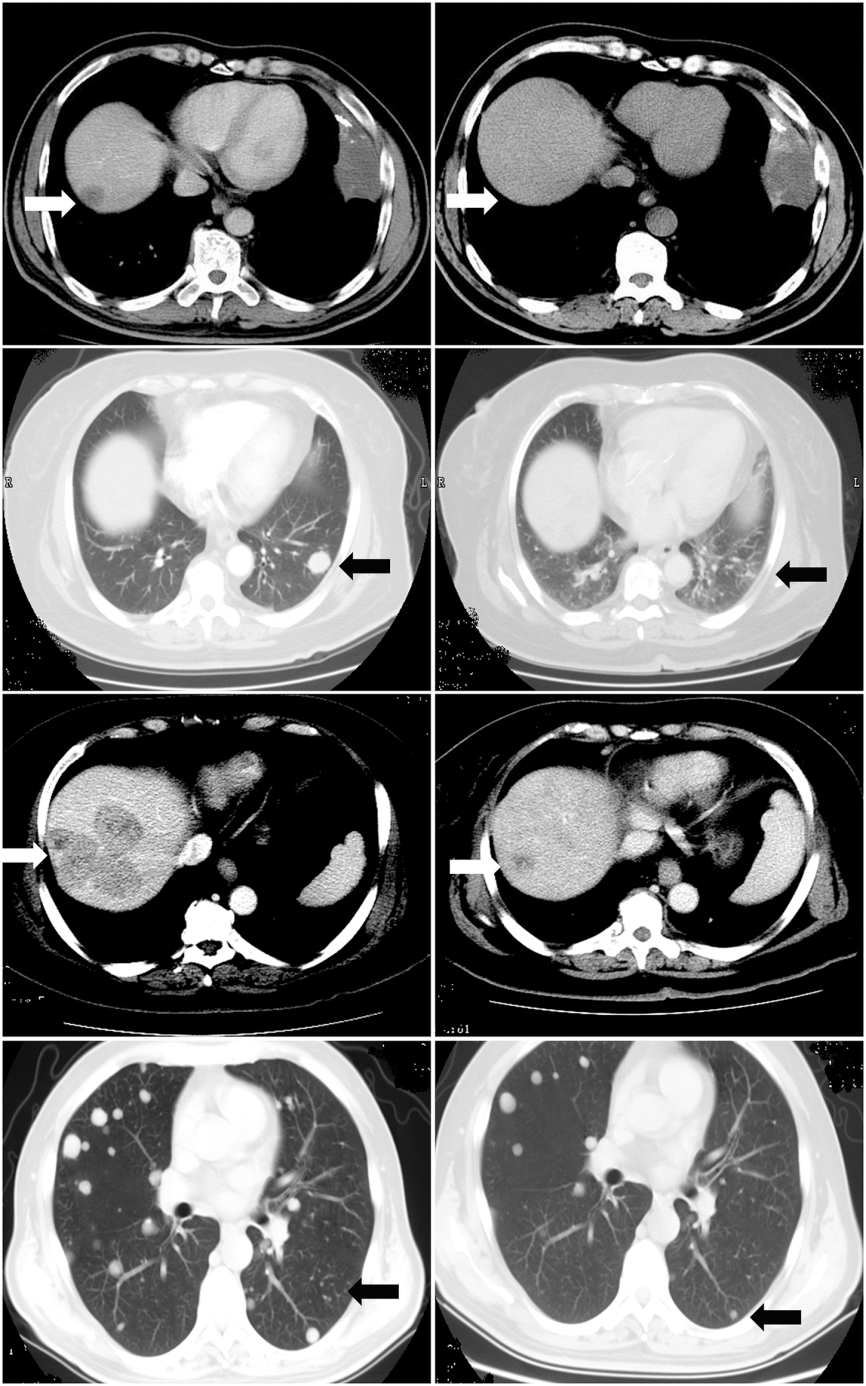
CT imaging of mCRC patients before and after treatment. Case 1 is a CR patient who was treated with four cycles of cetuximab plus irinotecan-based chemotherapy. Case 2 is a CR patient who was treated with three cycles of cetuximab plus oxaliplatin-based chemotherapy. Case 3 is a PR patient who received four cycles of cetuximab plus irinotecan-based chemotherapy. Case 4 is also a PR patient who was treated with four cycles of cetuximab plus oxaliplatin-based chemotherapy. CR, completely response; PR, partial response.
